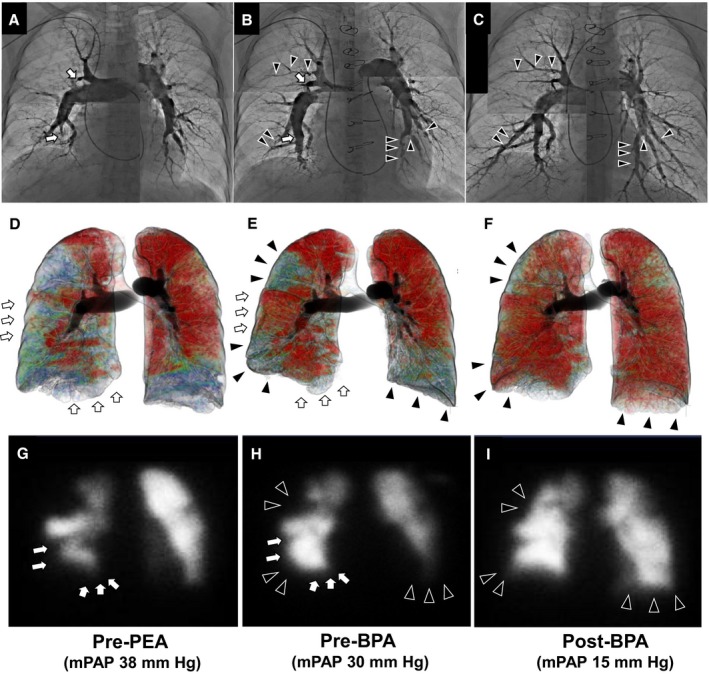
A representative case of hybrid therapy by additional BPA after PEA. Representative pulmonary angiography (A–C), dual‐energy CT (D–F), and lung ventilation–perfusion scintigraphy (G–I). PEA released the complete obstruction in the right anterior branch (right A3) and posterior basal branch (right A10), resulting in peripheral perfusion recovery in the area (white arrow). Four additional BPA sessions in the right peripheral lower lobe (right A8), upper lobe (right A3), and left lower lobe (left A8, A9, A10) improved the residual perfusion defects (black arrow). BPA indicates balloon pulmonary angioplasty; mPAP, mean pulmonary arterial pressure; PEA, pulmonary endarterectomy.
